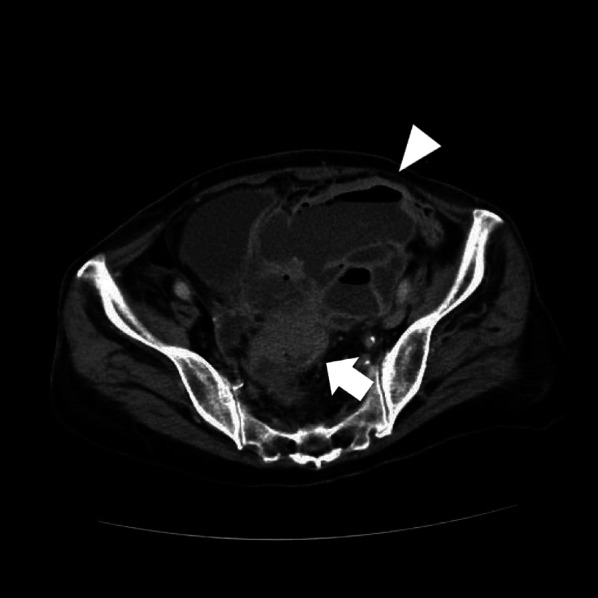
CT examination performed at the first visit. Sigmoid colon cancer (arrow) with a large intra-abdominal abscess (arrowhead) was suspected
